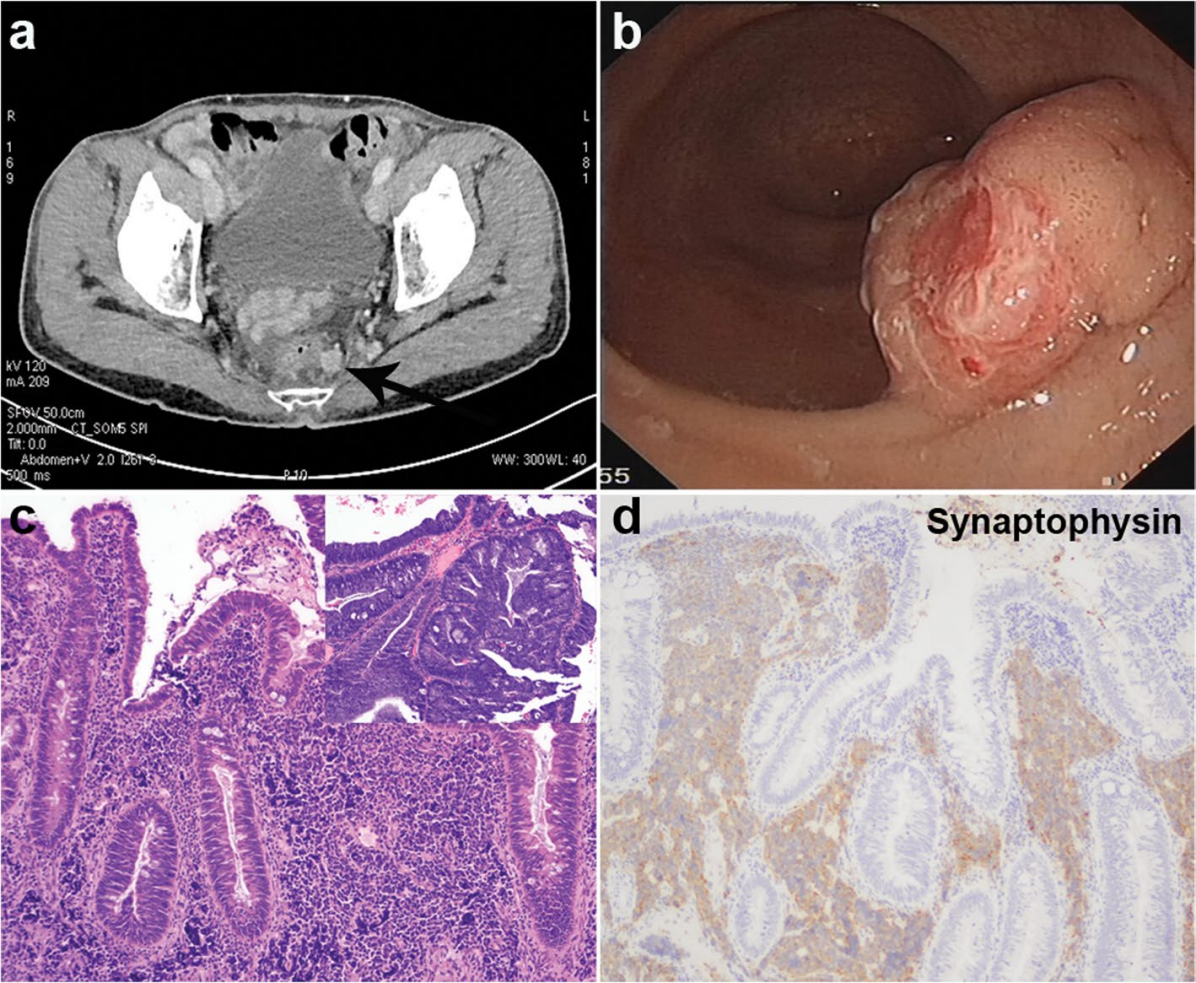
Representative images of the first operation. a CT of the abdomen revealed thickening rectal wall and swollen lymph nodes around the rectum. b Colonoscopy showed a 2.0 cm × 3.0 cm irregular bulge in the rectum. c The tumor exhibited a tubulovillous adenoma with high-grade dysplasia (upper right) on the surface and a component of SCNEC limited to the submucosa. d SCNEC was positive for synaptophysin
